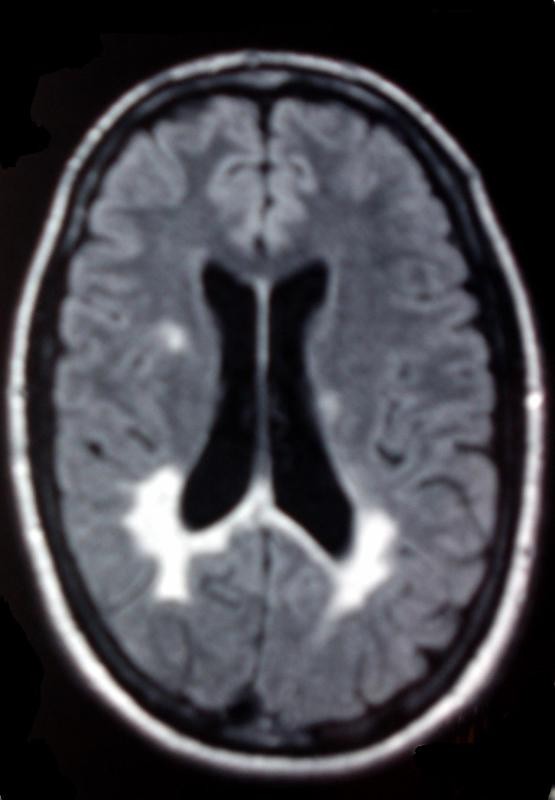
MRI at 5 year follow-up.
